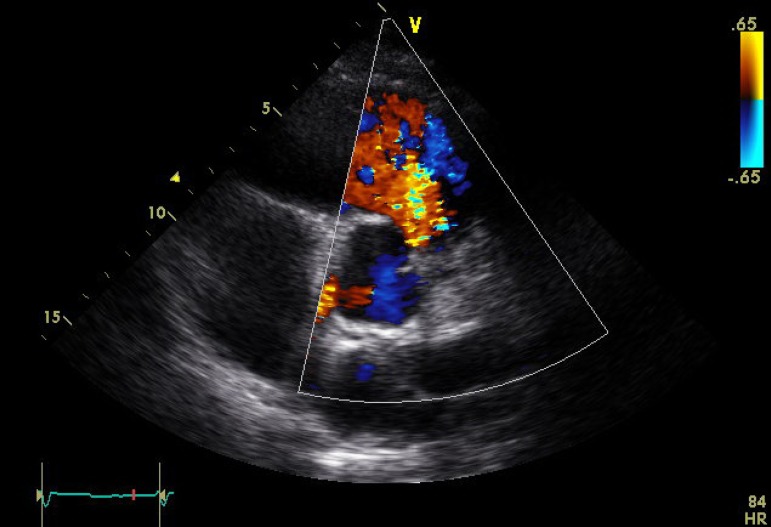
Transthoracic echocardiogram showing torrential pulmonary
